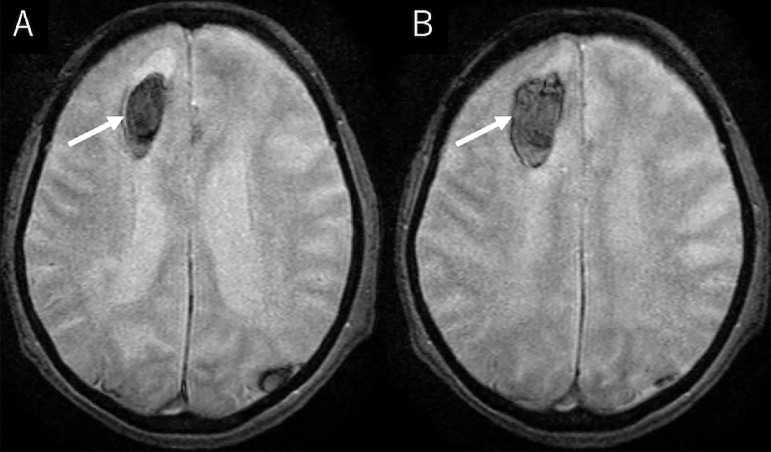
(A, B) Axial gradient-echo T2*-weighted magnetic resonance images showing the subcortical hemorrhage with no associated findings of cerebral contusion (*arrow*).
